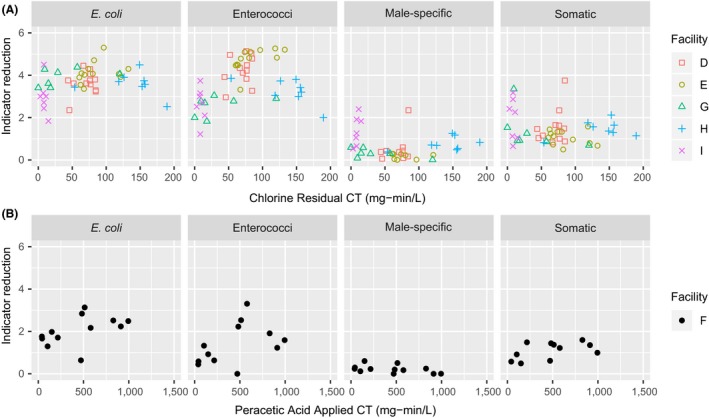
Bacterial and viral indicator disinfection reduction as a function of residual CT for chlorine (WRRFs D, E, G, H, and I) and applied CT PAA (WRRF F).
